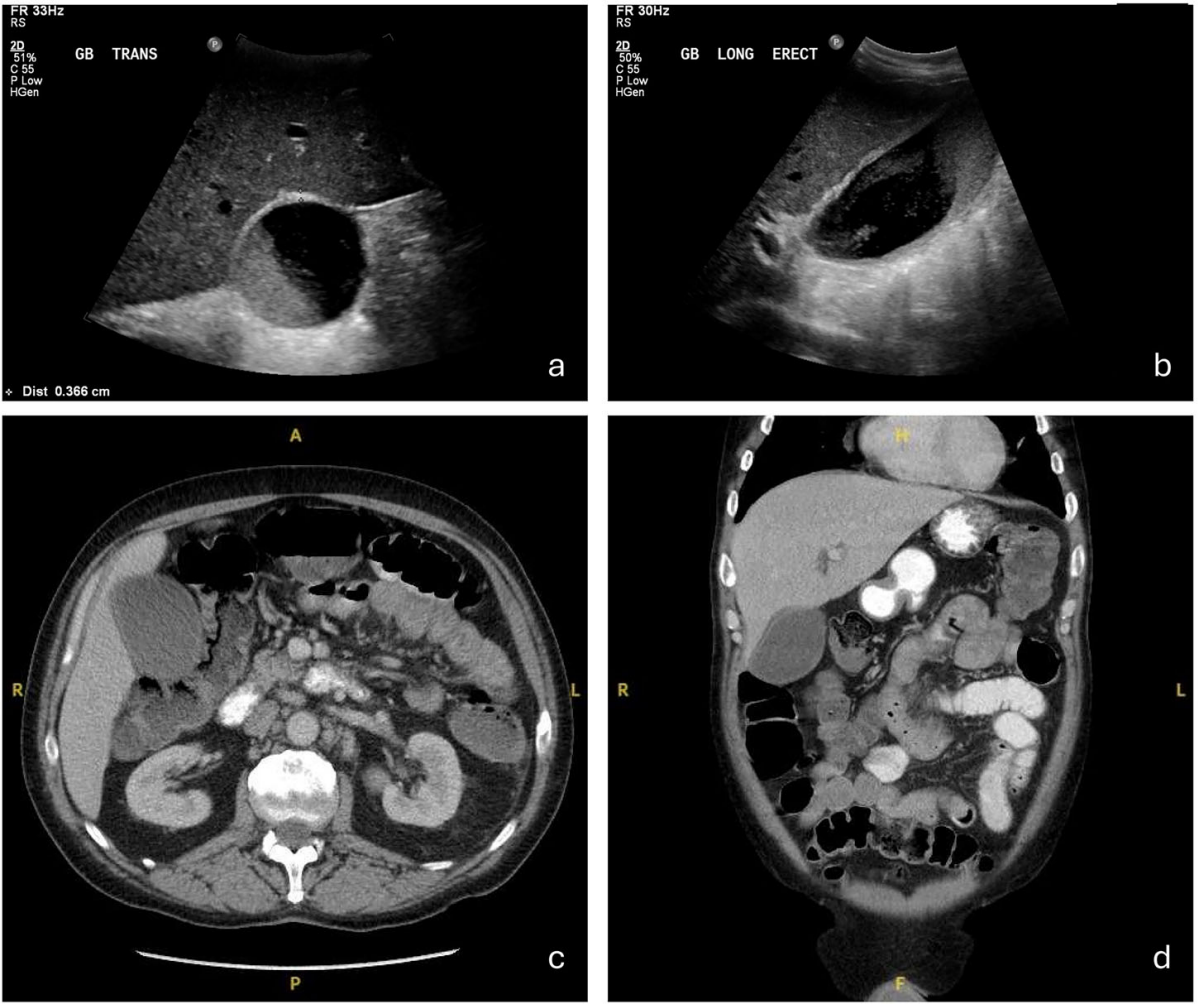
Fifty-nine-year-old male with suspected acalculous cholecystitis. He presented on day 10 post-chemotherapy with abdominal pain, rising inflammatory markers and a bilirubin of 60. Transverse (a) and longitudinal (b) views on US demonstrate gallbladder sludge and wall thickening of 3.6 mm. Axial and transverse planes on CT (c, d) show gallbladder distension, wall thickening, and no radiopaque stones. The patient subsequently underwent cholecystostomy, which was successful at preventing further complications
